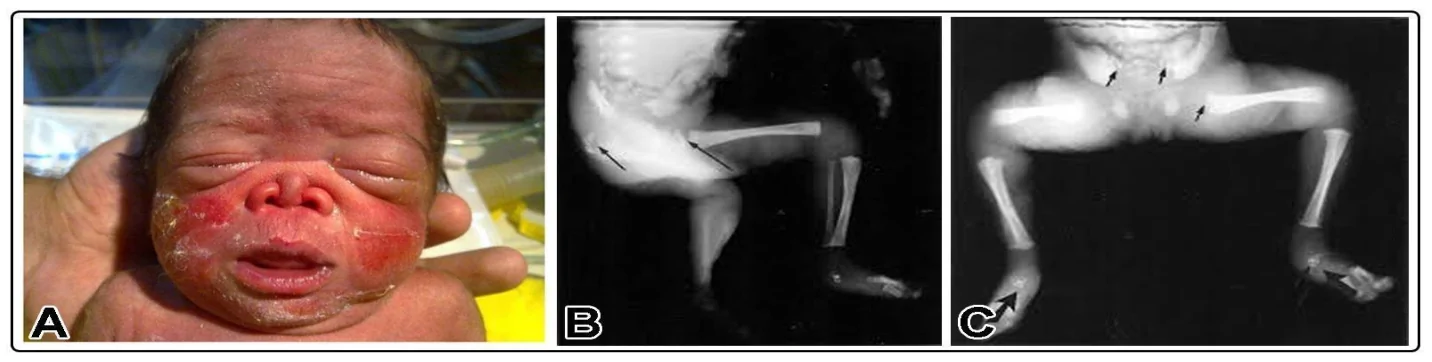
Photographic (A) and radiographic (B,C) representation of Warfarin Embryopathy. (A) Preterm newborn at 33 weeks of pregnancy delivered a male fetus with nasal hypoplasia characterized by a depressed nasal bridge and flat upturned nose (taken from Gupta et al., 2010) [43]. (B,C) Aborted fetus following termination of pregnancy at 23 2/7 weeks’ gestation, showing bilateral calcific stippling of the proximal femoral epiphyses (B, arrows), the iliac wings, and the tarsal bones (C, arrows) (taken from Chan et al., 2003) [31].
